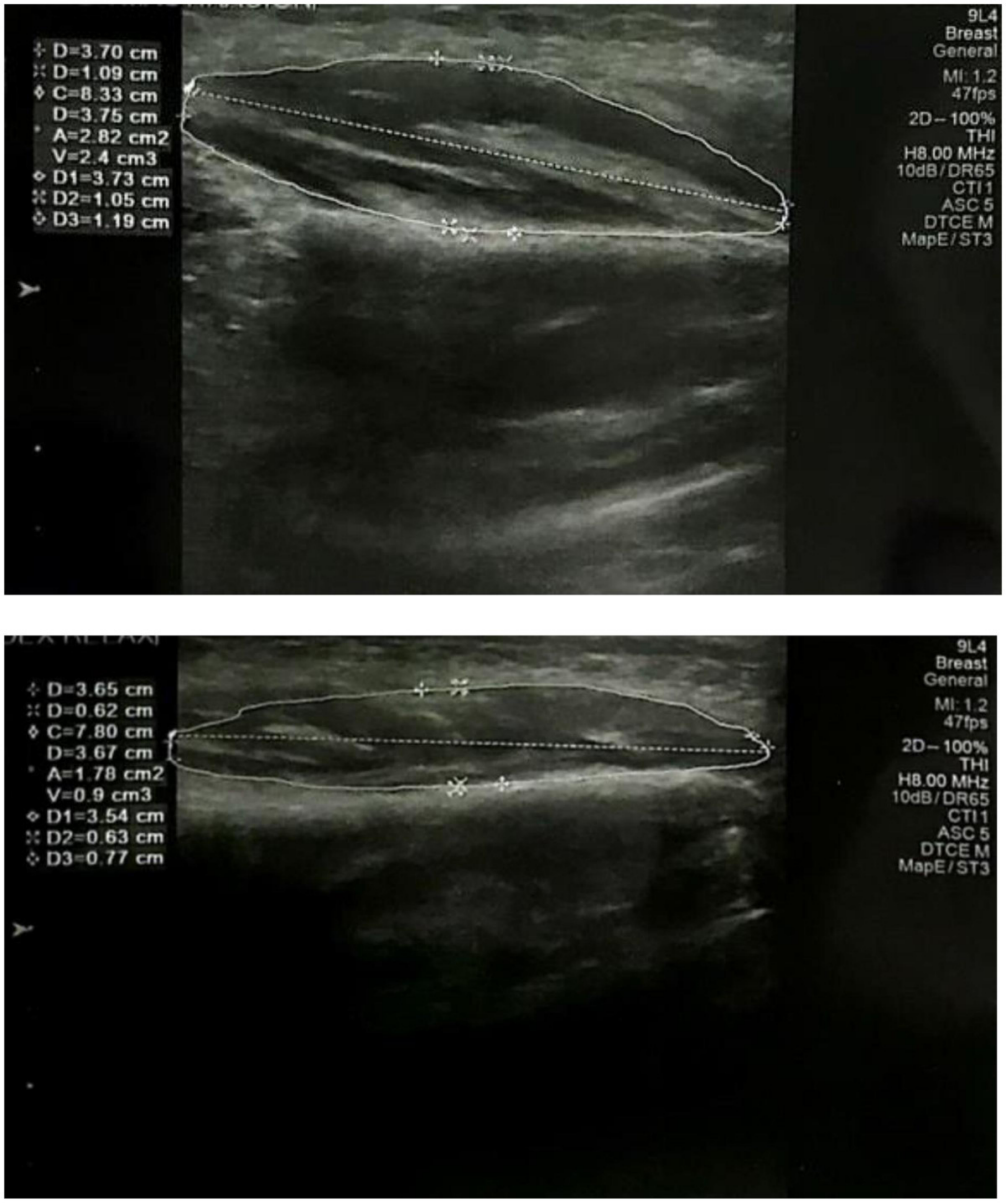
Ultrasound image of the masseter muscle.
